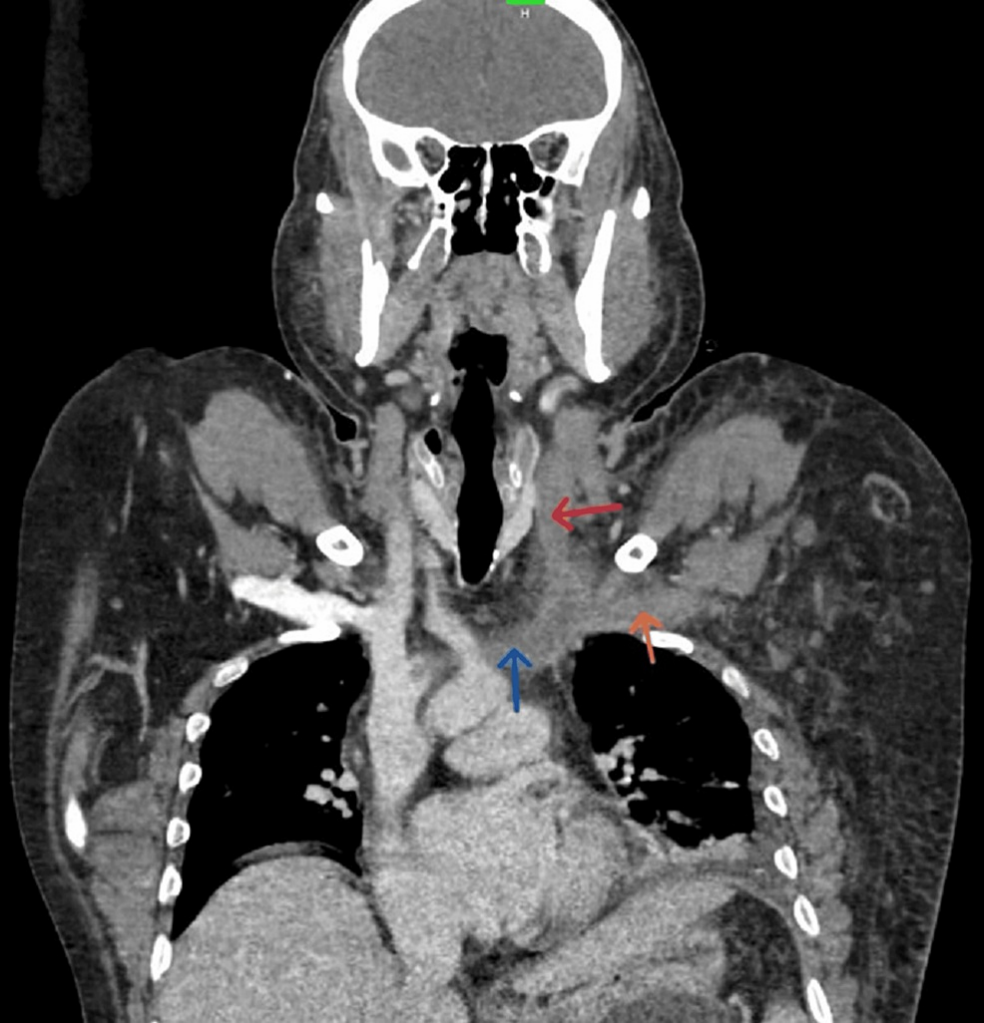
Coronal reformatted contrast-enhanced CT showing hypodense filling defects in left IJV (red arrow), left innominate vein (blue arrow), and left subclavian vein (orange arrow). Corresponding vessels on right show normal contrast opacification.
